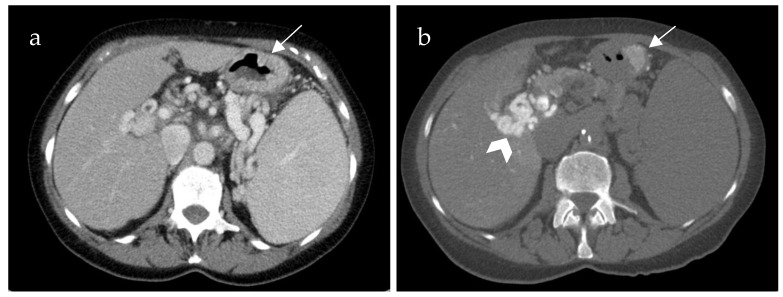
70-year-old female patient with portal hypertension due to chronic portal vein thrombosis. Axial images of contrast-enhanced computed tomography (CECT) (a) and computed tomography mesenterico-portography (CT-MPG) after contrast injection into the superior mesenteric artery (b). The CECT image shows diffuse contrast enhancement of the gastric wall (a) (white arrow). CT-MPG detects the partial hyperperfusion of the gastric wall (white arrow) and the cavernous transformation of the portal vein (white arrowhead (b)).
